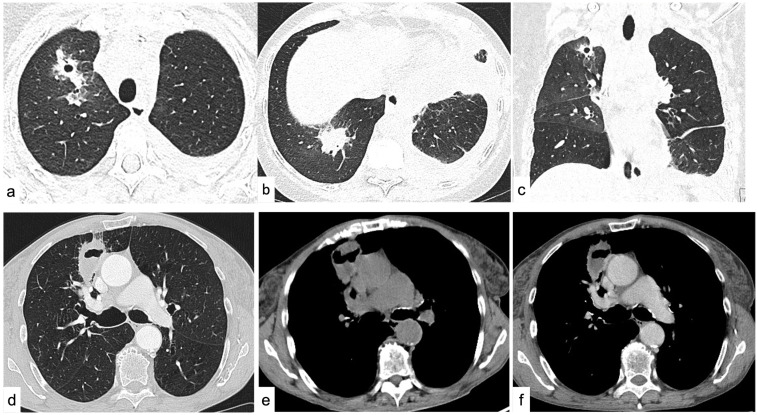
Pulmonary aspergillosis vs. cavitated metastases by squamous cell carcinoma of the tongue. Different cavitated lesions showing spiculated margins: pulmonary aspergillosis. Axial (a,b) and coronal (c) view of multiple solid cavitated nodules, with spiculated margins and peripheral ground glass (halo sign) in a 43-year-old neutropenic patient with follicular non-Hodgkin lymphoma (NHL), presenting with fever and cough. Cavitated metastases by squamous cell carcinoma of the tongue. Axial CT scans before (d,e) and after iodinated contrast medium administration (f) showing cavitated nodule in the paramediastinal site in a 67-year-old female patient with history of squamous cell carcinoma of the tongue.
